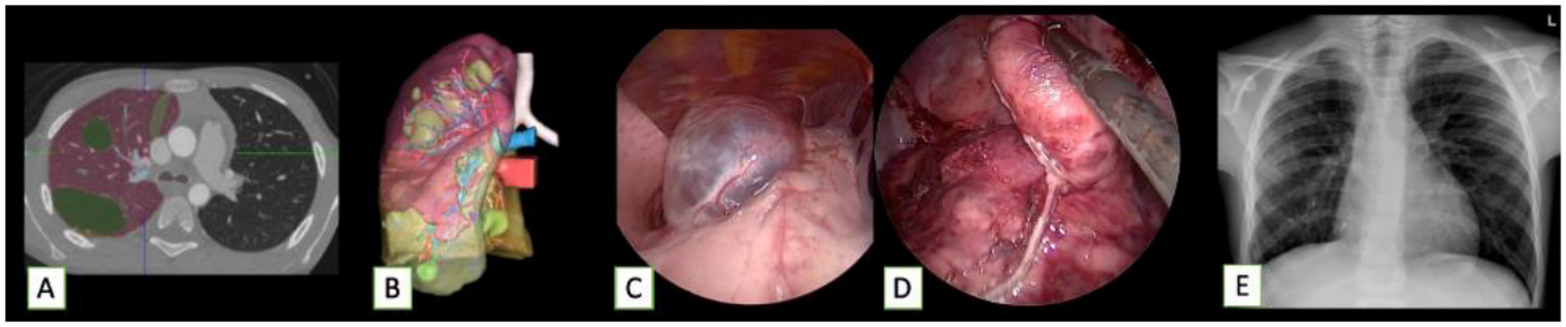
Cystic lesions after surgery and chemotherapy for a pleuropulmonary blastoma in a 7-year-old boy, who then underwent MIS excision of multiple masses. (A,B) Reconstruction and 3D model of the lesions. (C,D) Intraoperative thoracoscopic view. (E) Post-operative X-ray.
